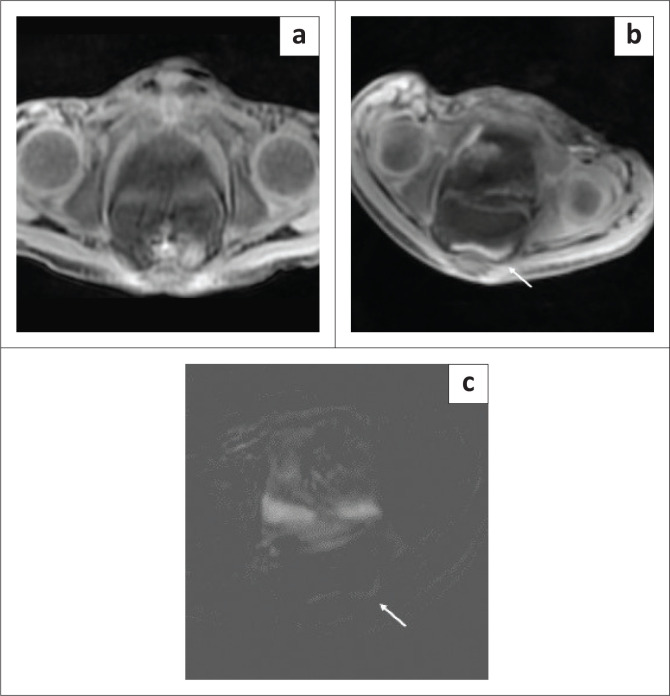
Case 2 (a) axial pre-contrast T1-weighted image of the pelvis and (b) axial T1-weighted image of the pelvis 12 min after intranodal contrast administration depicted leakage of contrast in the pelvic free fluid (arrow). (c) The leak was highlighted in the subtracted image (arrow).
